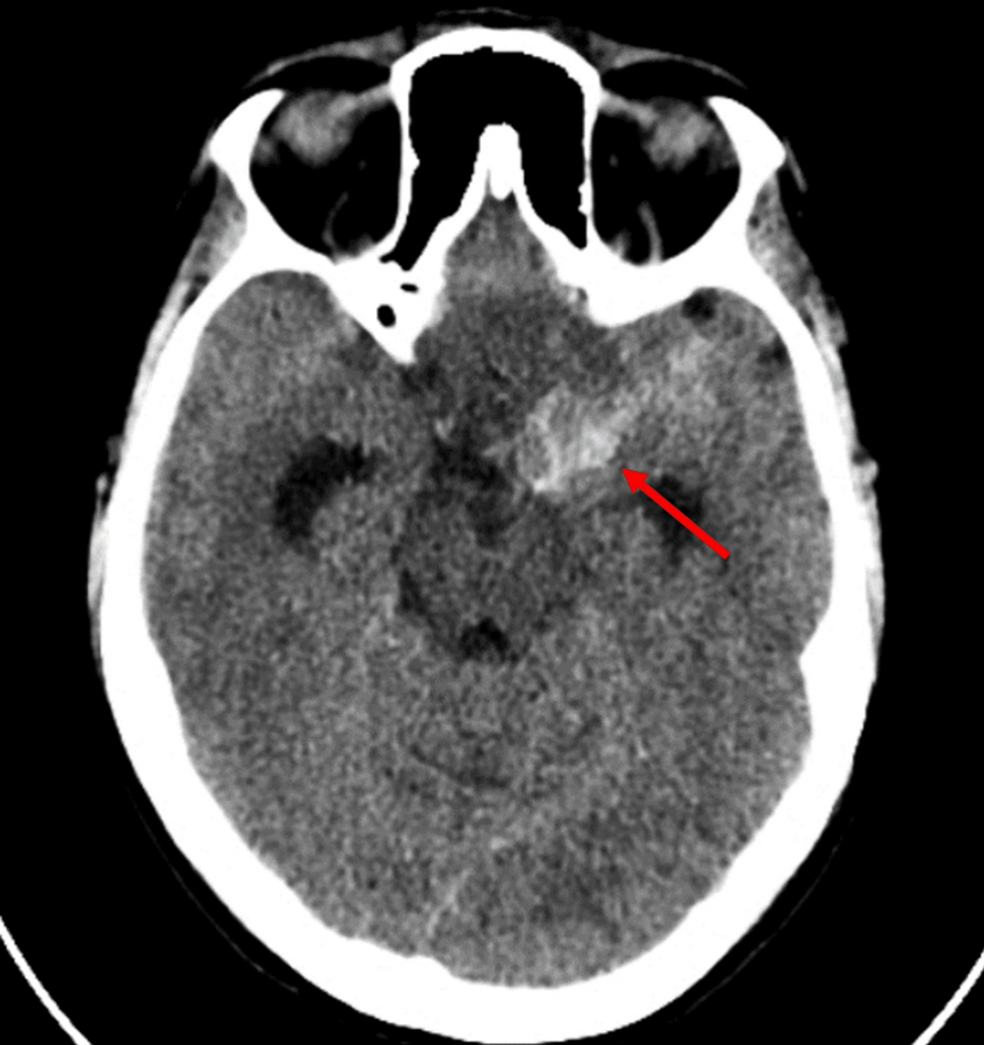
Brain CT Diffuse subarachnoid haemorrhage (arrow) in the basal cistern, predominantly on the left side of the pentagonal cistern
